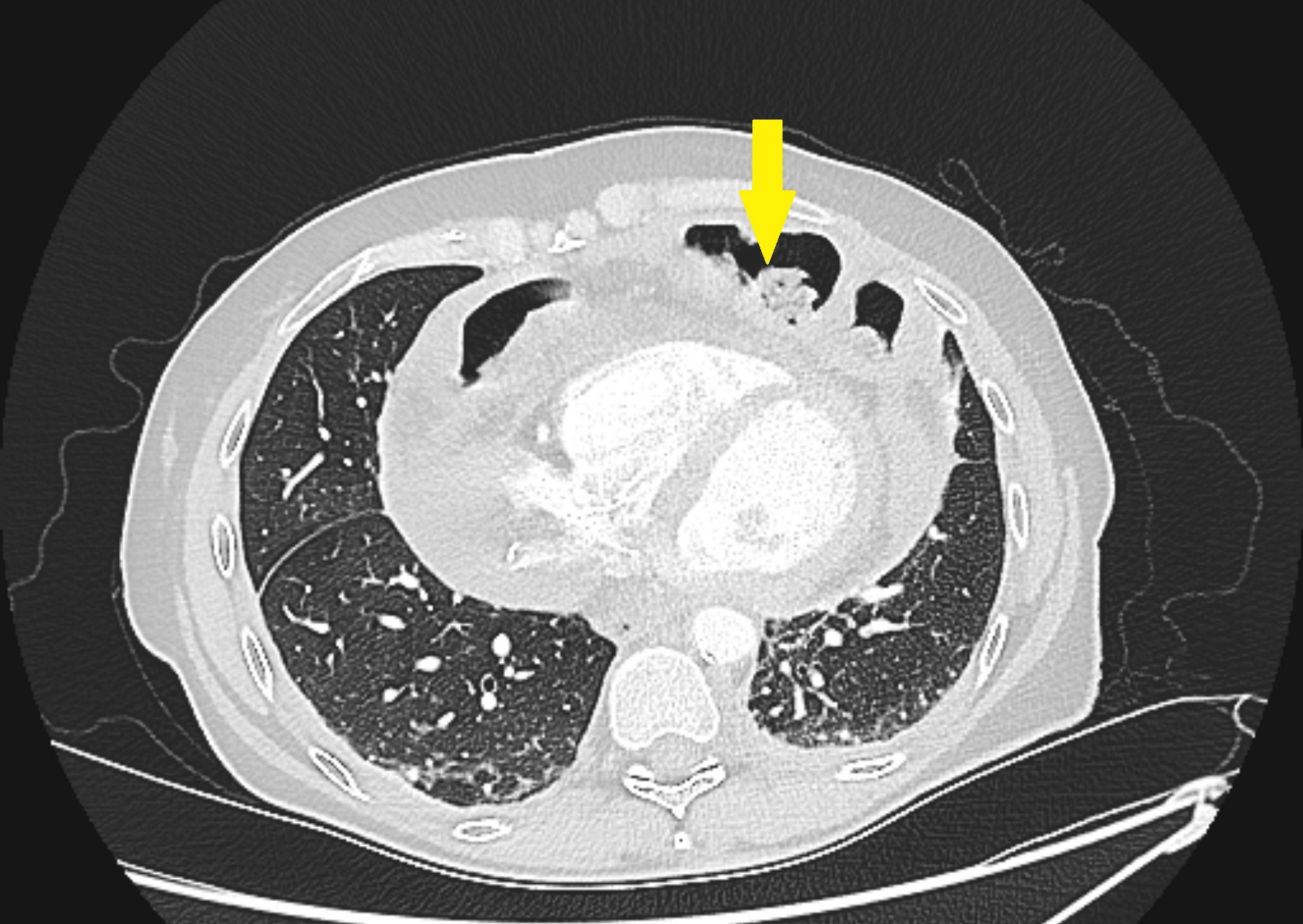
CT axial view showing transverse colon (yellow arrow) anterior to the heart within the pericardial sac
